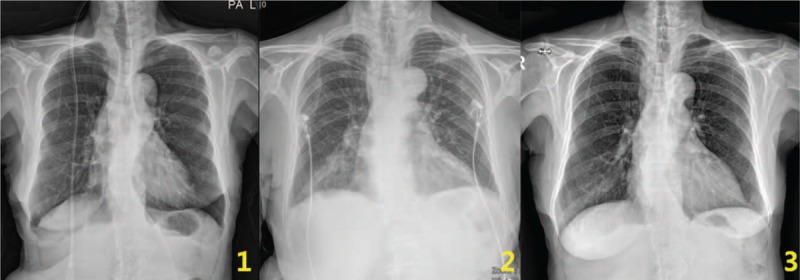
Chest X-ray. (1) No remarkable finding (April 20, 2017). (2) Bronchopneumonia at the right lower lung field (April 23, 2017). (3) No active lung infiltrations (June 7, 2017).
